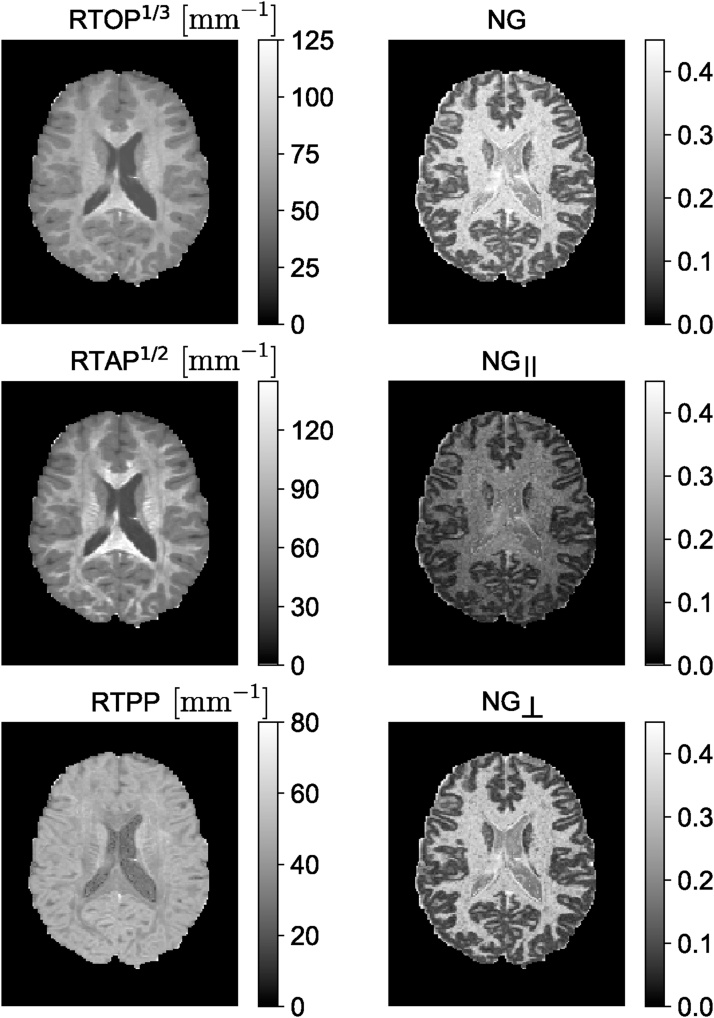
MAP-MRI indices of an HCP WuMinn data with *b* = 1000 , 2000, , and 3000 s/mm^2^. RTOP – return-to-the-origin probability, RTAP – return-to-the-axis probability, RTPP – return-to-the-plane probability, NG – non-Gaussianity.
